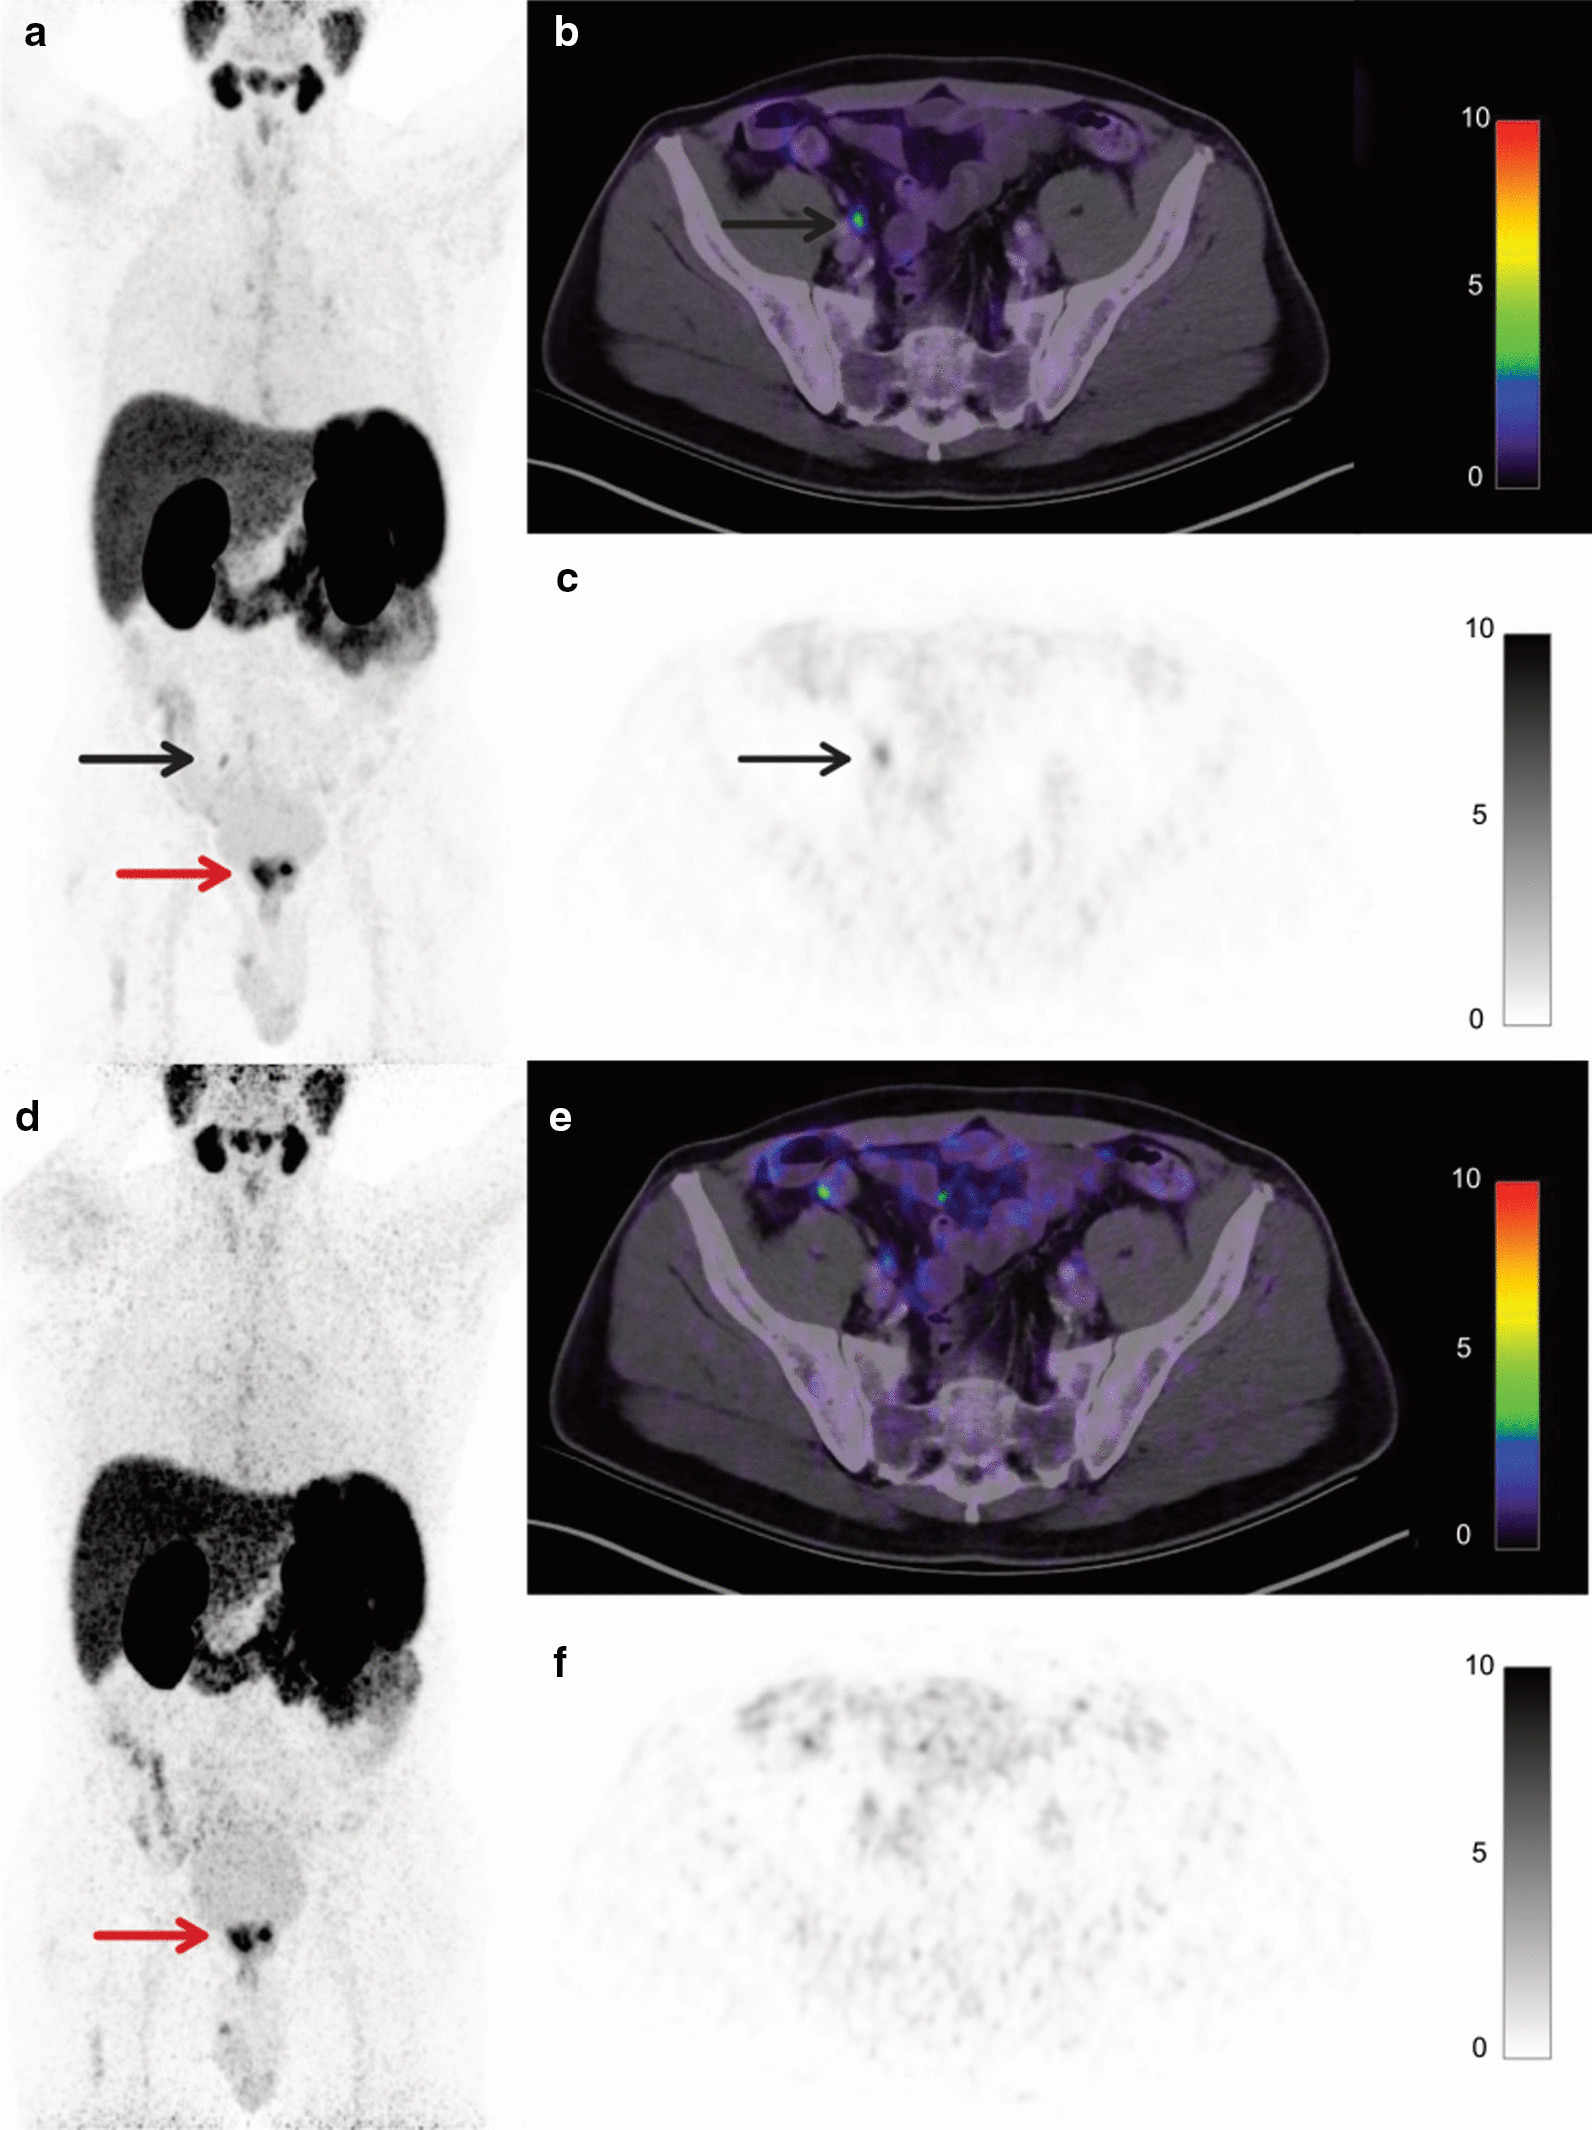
A 61-year-old patient (Pat ID #13) with biopsy-proven prostate cancer undergoing [^68^Ga]Ga-PSMA-11 PET/CT for initial tumor staging before treatment. Gleason Score was 9, PSA was 18.3 ng/ml at the time of imaging. a–c show images acquired with the reference acquisition protocol; d–f show images acquired with the reduced acquisition protocol all reconstructed with OSEM-PSF + TOF(4i). Maximum intensity projection (a, d) and axial [^68^Ga]Ga-PSMA-11 PET/CT slices (b, c, e, f) show local tumor (red arrows). One right pelvic lymph node metastasis (black arrow) could not be unequivocally detected with a three and a half-fold scan time reduction (c, f). Until now the patient has not undergone surgery, a follow-up scan performed more than 6 months later confirmed the prostatic and lymph node lesions
